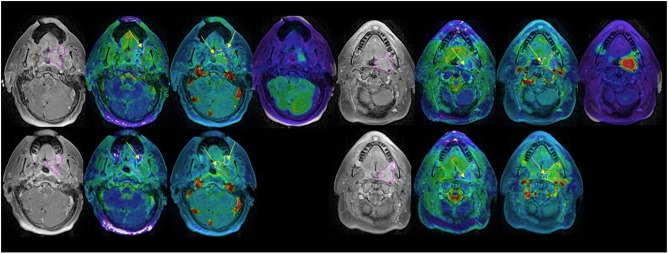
Post Gd T1 weighted images (left), ADC (second left), blood volume (second right), and SUV (right) FDG PET pre-RT (top) and after 10 fractions of radiation therapy (bottom). GTV: magenta; low BV subvolume: yellow; low ADC subvolume: cyan; MTV: red. Note persistent low BV and low ADC subvolumes after 10 fractions of radiation therapy (yellow arrows).
